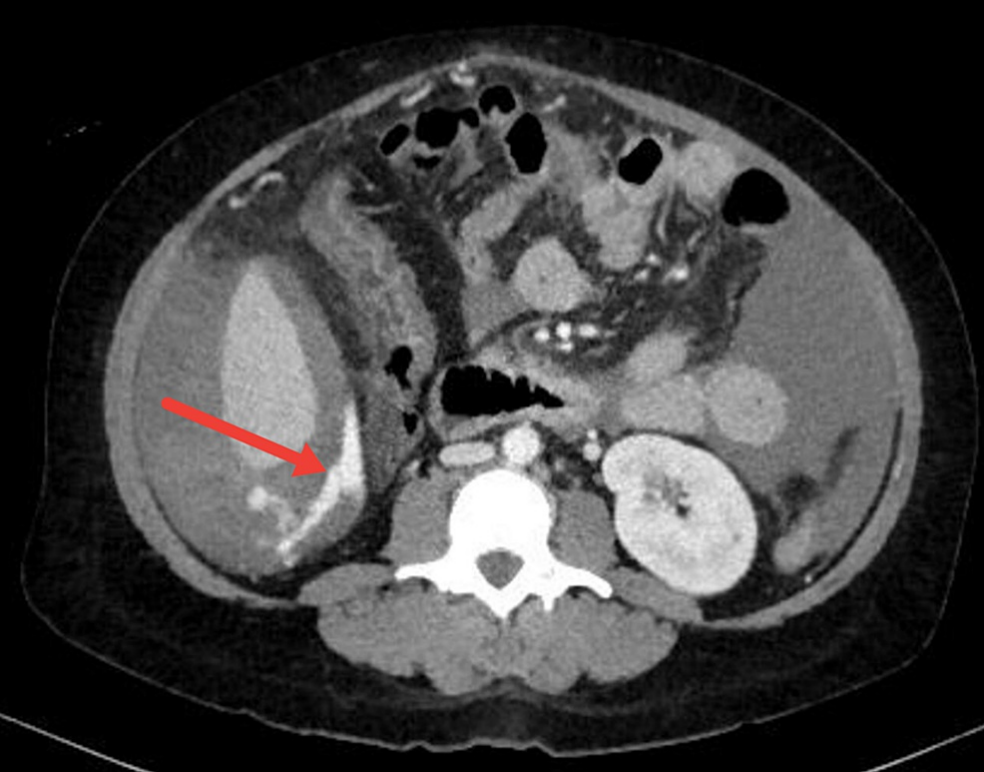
Axial CT image demonstrates an active extravasation of blood inferior to the right hepatic lobe (arrow). CT, computed tomography.
